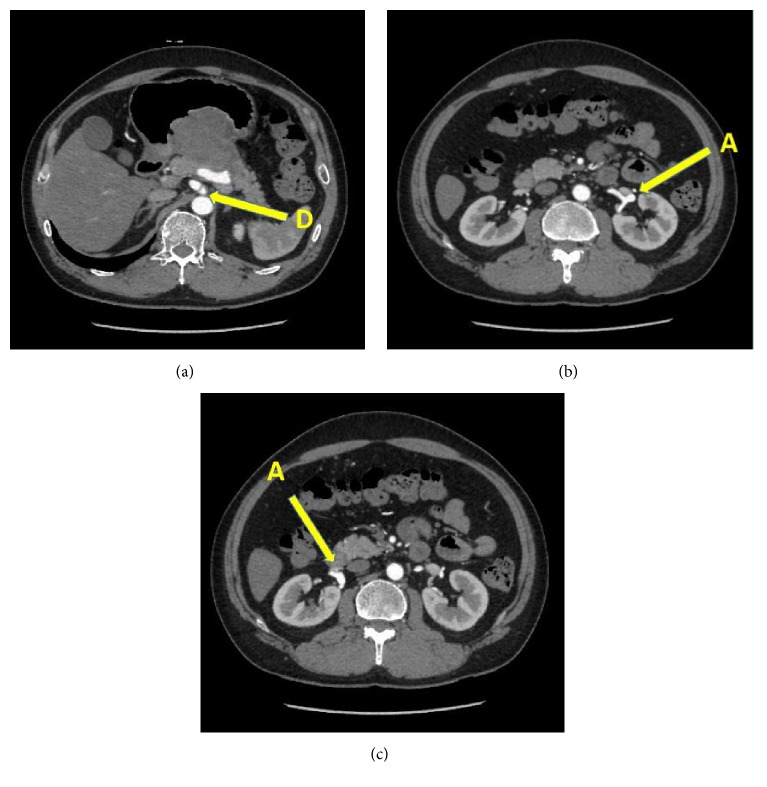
CT angiogram indicated dissection of common hepatic artery as well as aneurysms of the common hepatic artery (maximum diameter, 8 mm) and bilateral renal artery (maximum diameter, 5 mm). D: dissection; A: aneurysm.
